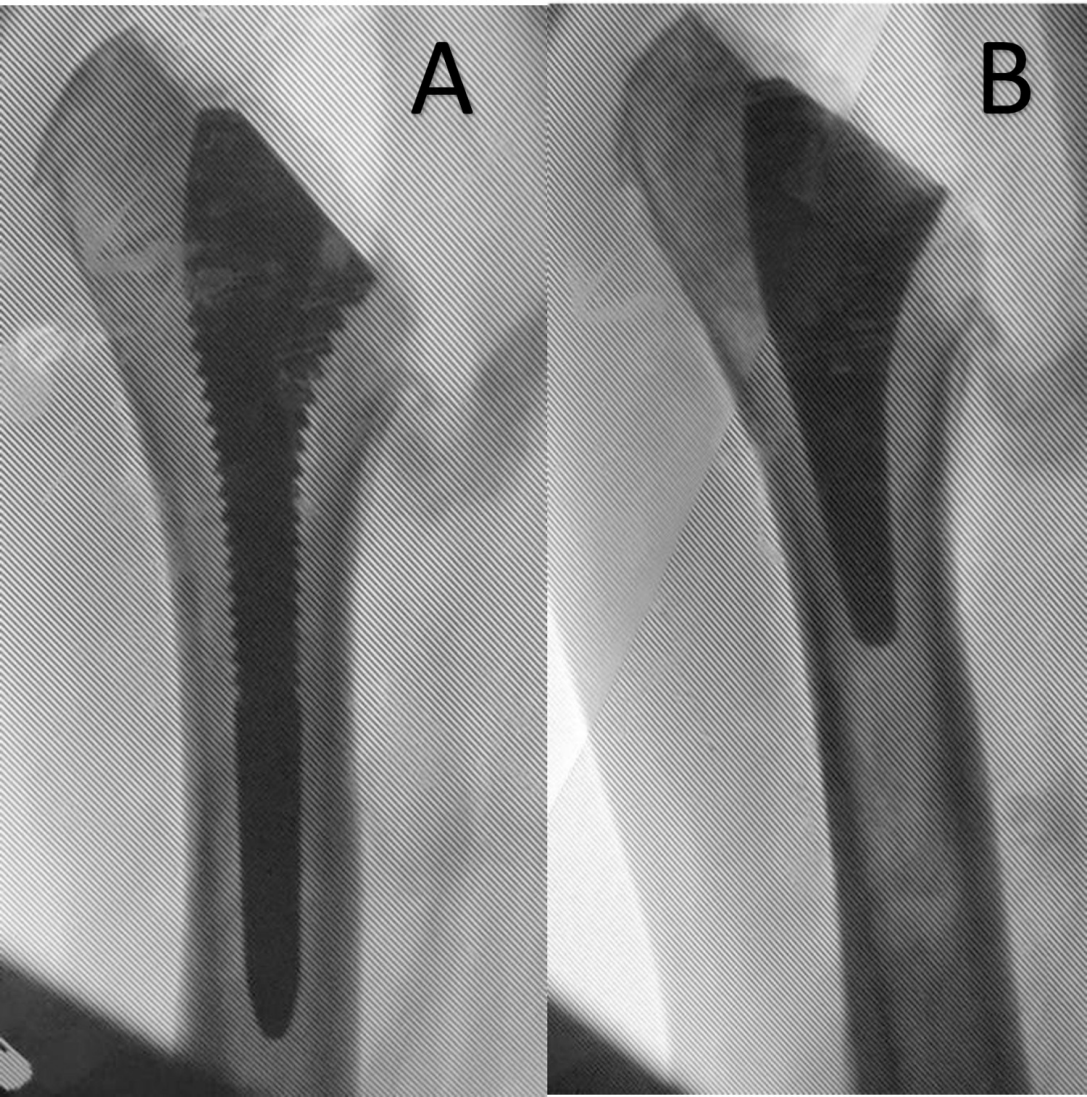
True anteroposterior humeral radiographs showing well aligned implants (size 7), without cortical contact (A) trial (alpha angle 0,5°), (B) short-stem (alpha angle 0,1°), in the same subject.
